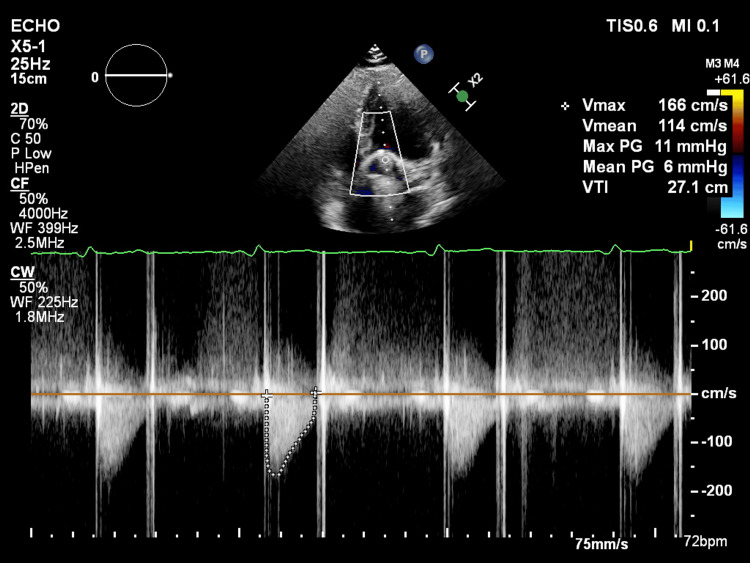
Postoperative TTE With Continuous Wave Doppler Showing Normal Prosthetic Valve Hemodynamics. The upper panel shows two-dimensional imaging demonstrating the prosthetic valve in the aortic position with characteristic acoustic shadowing. The lower panel displays the continuous wave Doppler spectral tracing across the prosthetic valve, revealing excellent hemodynamic performance with a peak velocity (V_max_) of 166 cm/s, mean velocity (V_mean_) of 114 cm/s, peak pressure gradient of 11 mmHg, mean pressure gradient of 6 mmHg, and velocity time integral (VTI) of 27.1 cm. The smooth Doppler envelope without turbulence indicates normal prosthetic valve function with no evidence of stenosis, patient-prosthesis mismatch, or significant regurgitation. These findings represent a dramatic improvement from preoperative values (peak gradient 79 mmHg, mean gradient 42 mmHg) and confirm successful mechanical aortic valve replacement with optimal hemodynamic outcomes. TTE: transthoracic echocardiography
